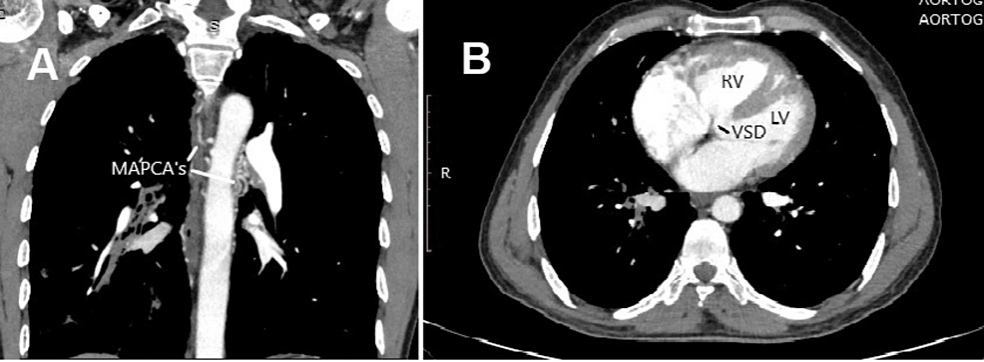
Cardiac computed tomography showing (A) multiple aortopulmonary collateral arteries (MAPCAs), (B) ventricular septal defect with overriding of the aorta, right ventricular hypertrophy. RV = right ventricle, LV = left ventricle, VSD = ventricular septal defect
